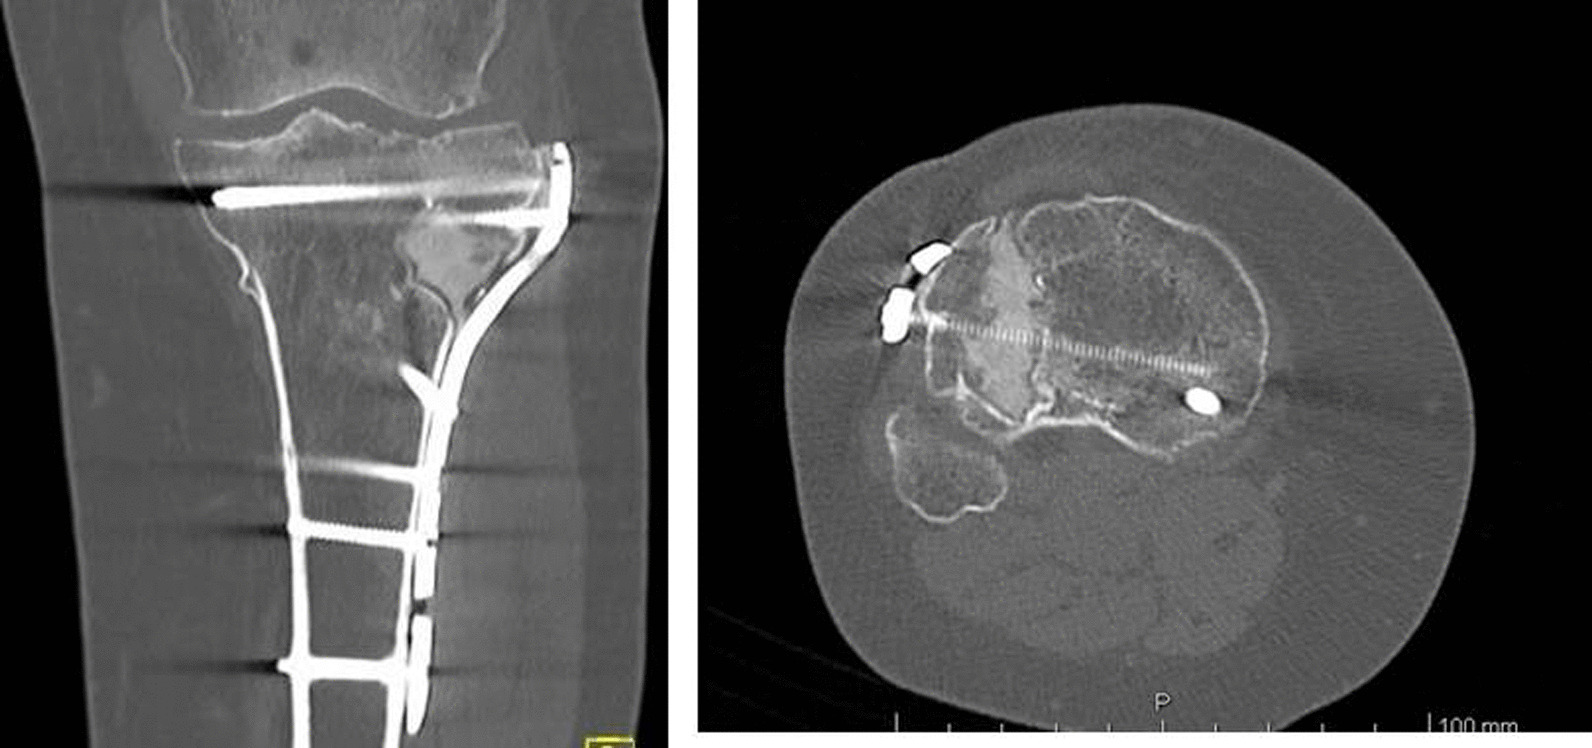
One-year postoperative CT scan demonstrates a partial surface integration without radiolucent lines or osteolysis with good evidence of interdigitation and even osteointegration
